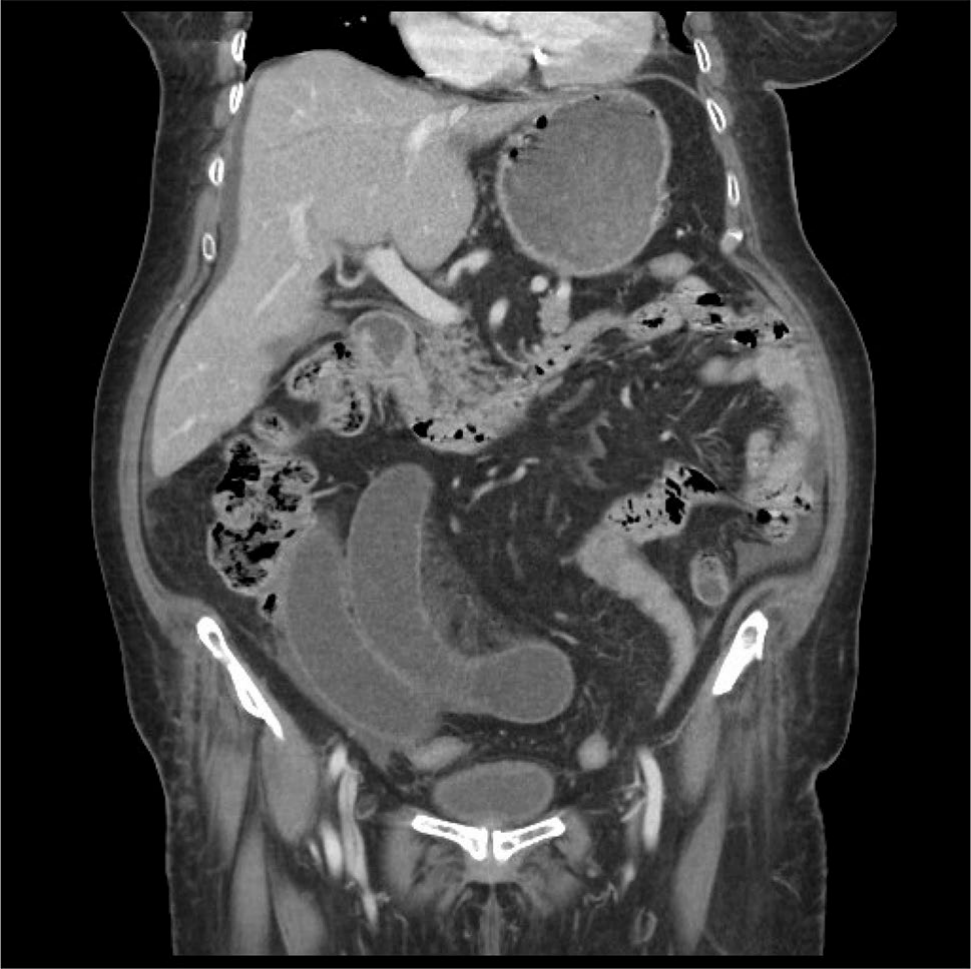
CT abdomen with contrast showed dilated small bowel loops in the right side of the lower abdomen and pelvis with a short segment critical stenosis measuring about 2.3 cm
